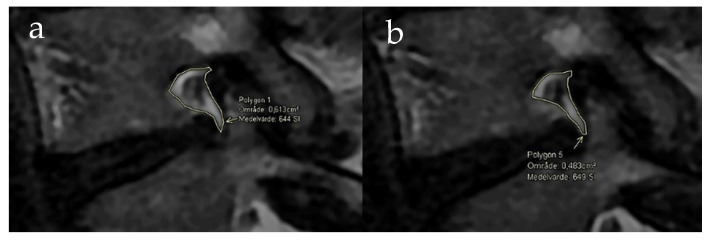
MRI of a right L4–5 foramina without loading of the spine (a) and MRI of the same foramina acquired with axial loading (b). The measurement area is marked in both images and a reduction in the area was measured. The fat surrounding the nerve root is visually reduced in the MRI with loading.
